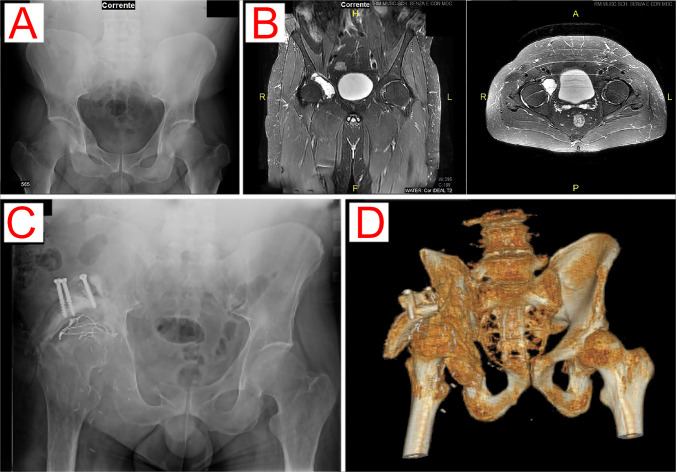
Fifty-three-year-old male patient with Grade 2 CS of the acetabulum (Enneking and Dunham’s area 2); preoperative X-ray (A) and STIR-MRI (B) highlights an acetabular osteolytic area with widening of the bone profile; C and D) postoperative X-ray and CT scans
